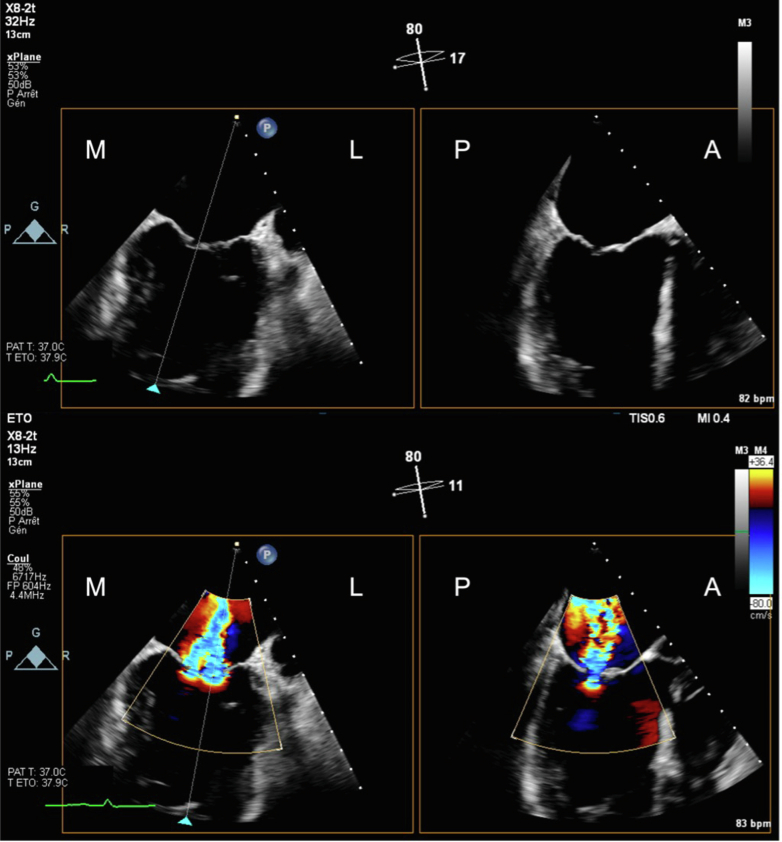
Preprocedural Transesophageal Echocardiographic Findings A = anterior; L = lateral; M = medial; P = posterior.
